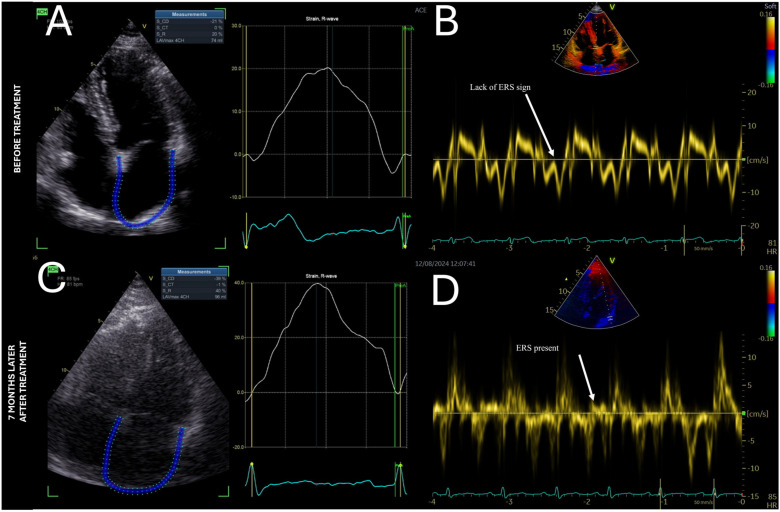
Echocardiographic imaging showing improvement in diastolic function after treatment. (A) Speckle tracking of the left atrial walls showed a peak strain rate of 20%, which proves second-degree diastolic dysfunction. (B) Tissue Doppler imaging of the IVS shows no ERS, indicating diastolic dysfunction. (C) After treatment, LA strain increased significantly to 40%. (D) ERS starts to be visible on the lateral wall.
